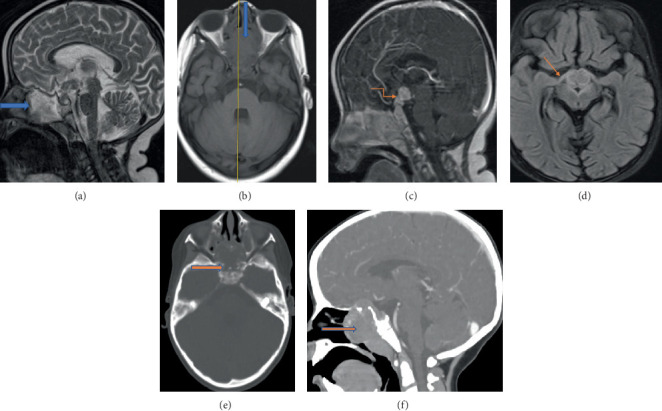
(a) There is a heterogeneously hyperintense lesion in the sphenoid sinus on sagittal T2W, (b) with intermediate signal intensity on T1W, associated with a nodular intermediate signal intensity lesion involving the pituitary, infundibulum, and hypothalamus. (c) Both the sphenoid sinus and intracranial sellar and suprasellar components show homogeneous contrast enhancement on sagittal T1+C. (d) There is optic tract and optic chiasm edema on axial FLAIR. (e) Axial CT scan in the bone window shows midline basal skull destruction and otomastoid air cell opacification, (f) while the sphenoid sinus and intracranial soft tissue components demonstrate enhancement on the soft tissue window of sagittal CT MPR (f).
